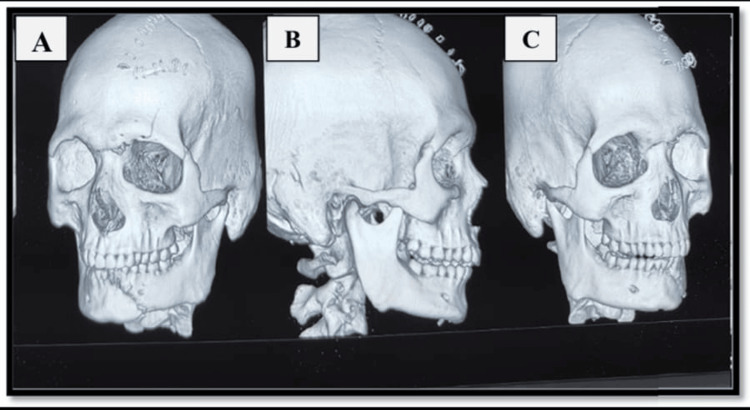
Preoperative three-dimensional (3D) CT of patient with displaced fracture of left parasymphysis of mandible: A) left oblique view; B) right view; C) right oblique view
